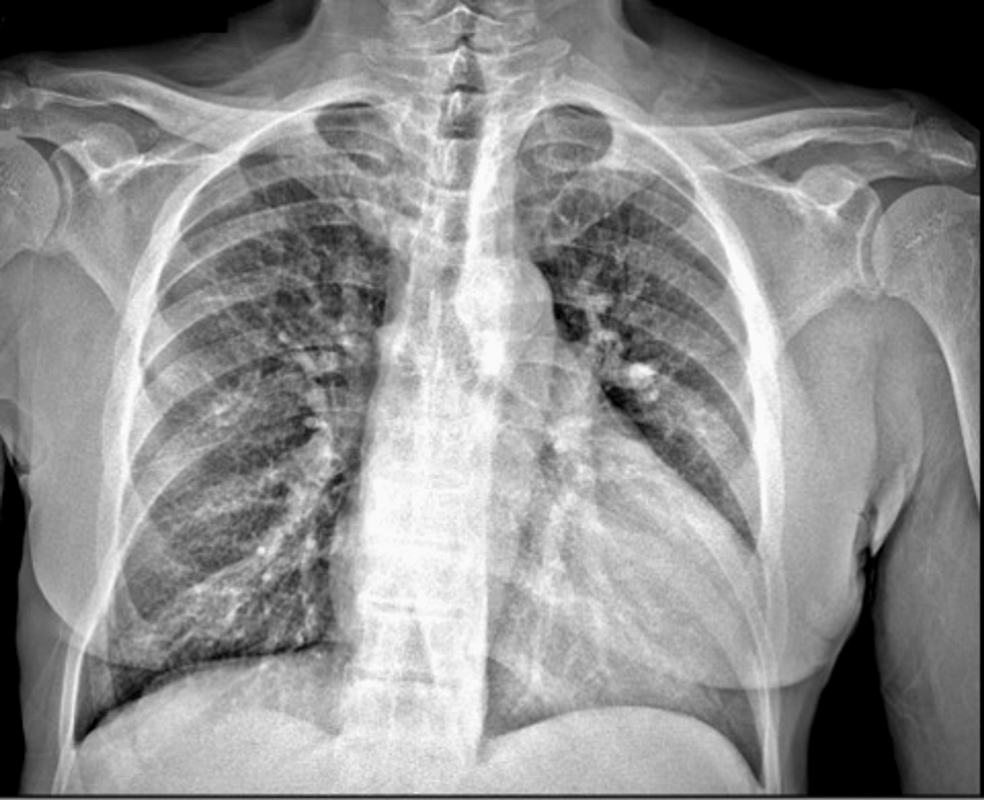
Chest x-ray showing cardiomegaly with non-specific perihilar prominence.
